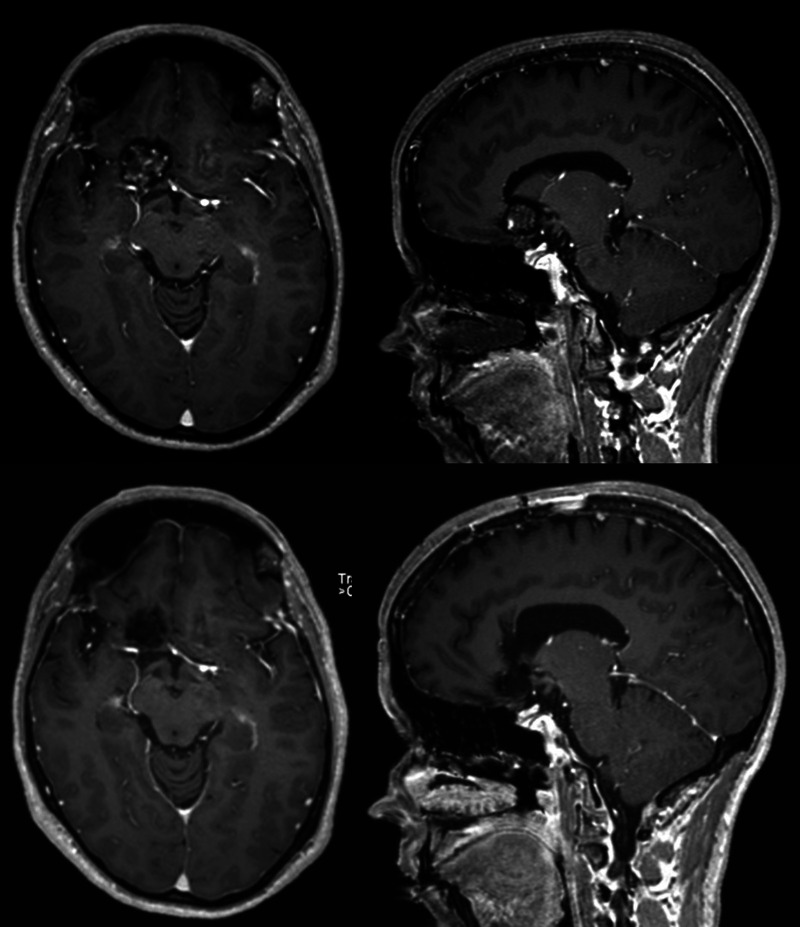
Brain MRI T1-post-gadolinium comparison between before (top) and after (bottom) surgical resection of a right subcallosal cavernoma.
